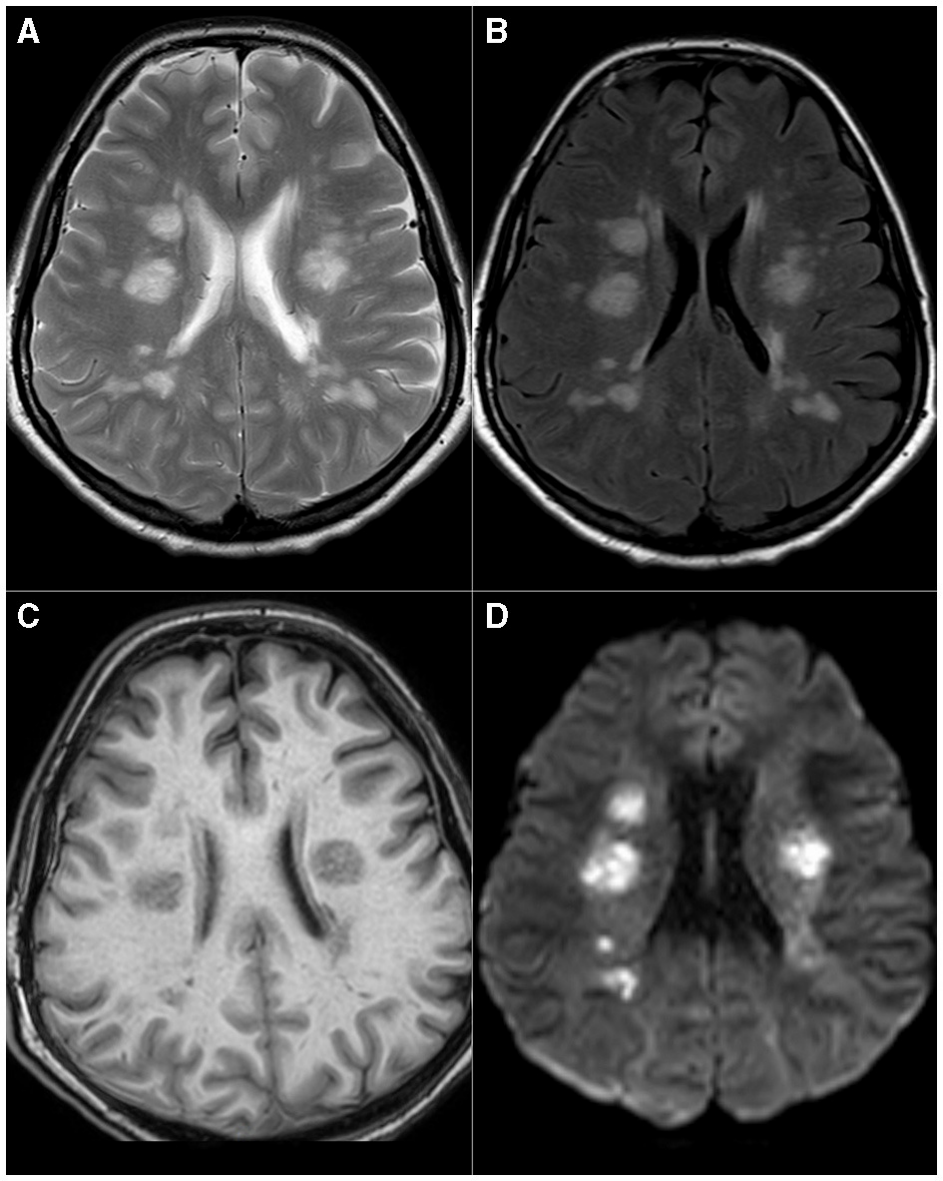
Acute disseminated encephalomyelitis (ADEM). Multiple, bilateral T2 (A) and FLAIR (B) hyperintense foci present in periventricular areas of white matter, hypointense, or invisible on T1-weighted images (C). These lesions show pronounced diffusion restriction seen as high-signal intensity on DWI images (D).
